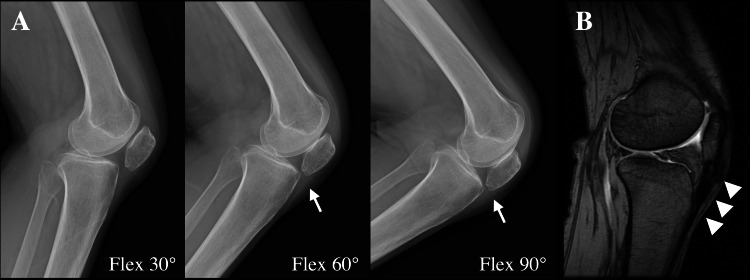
Preoperative imaging findings Lateral knee radiographs revealed progressive patella baja during flexion, with patellar impingement on the proximal tibia at 60° flexion (A, arrow). MRI showed a high-intensity signal in the proximal portion of a thickened patellar tendon (B, arrowhead).
